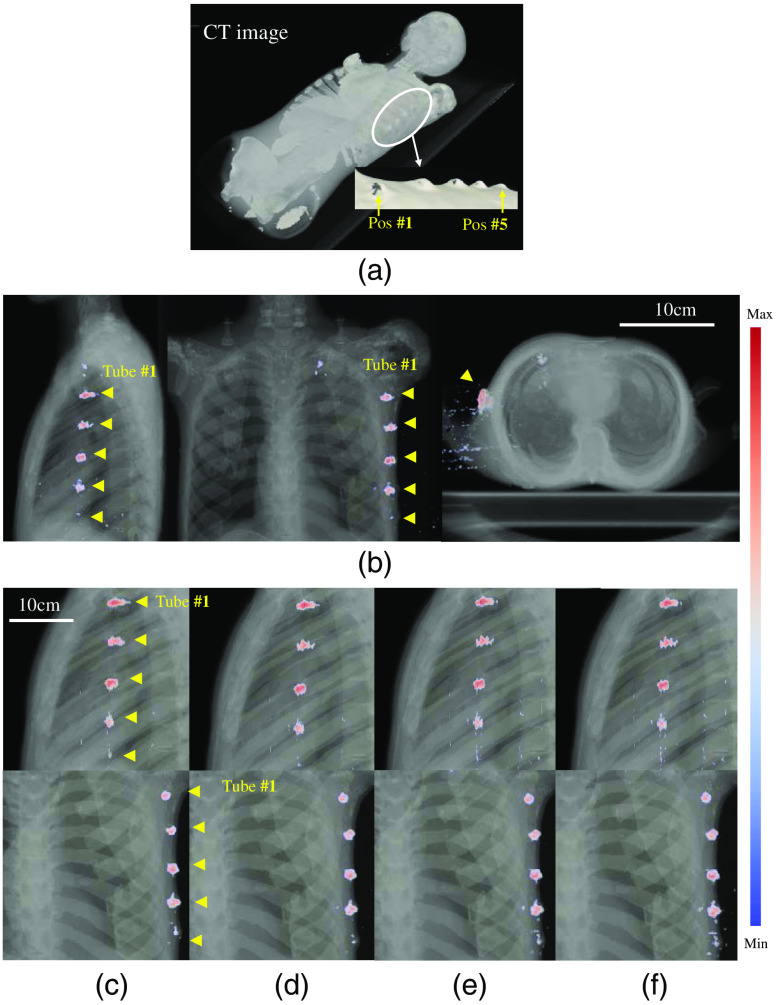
3-D images of (a) CT and (b) overlaid with CELSI for three orthogonal views: coronal, sagittal, and transverse. Compositions of CT and CELSI images for varying numbers of light sheets, which correspond to less dose delivery. In these images, the number of sheets were: (c) 25, (d) 50, (e) 100, and (f) 250, respectively.
